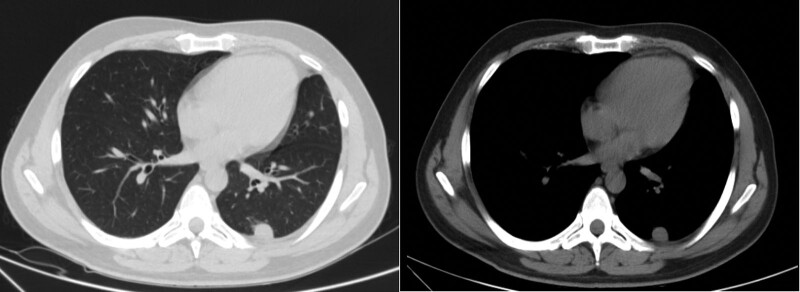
CT scans of patient: (A) and (B) pulmonary metastasis. CT = computed tomography.
